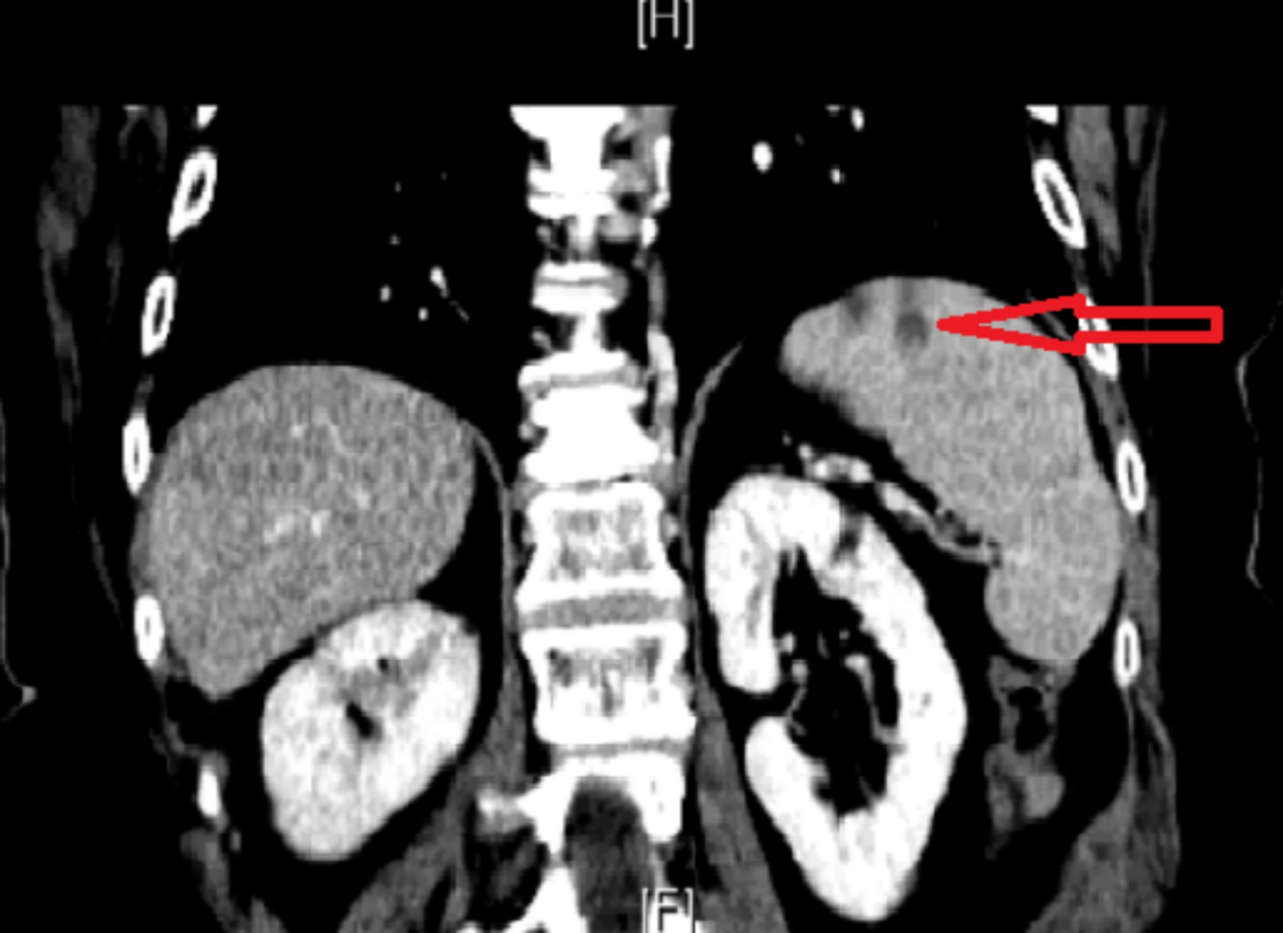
Computed tomography (CT) scan of the abdomen showing the splenic infarcts
